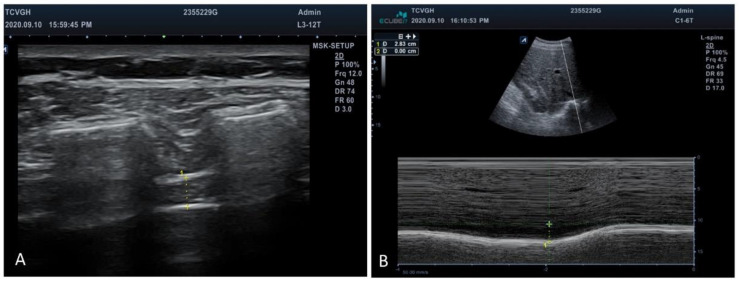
Ultrasonographic evaluation of diaphragm. (A) Diaphragmatic thickness was measured below the intercostal muscles between the ribs. +: Markers of the anterior and posterior edges of diaphragm. (B) Amount of diaphragmatic excursion was measured using the M mode to trace the movement of the posterior edge of liver. +: Markers of the posterior edge of liver during respiration.
